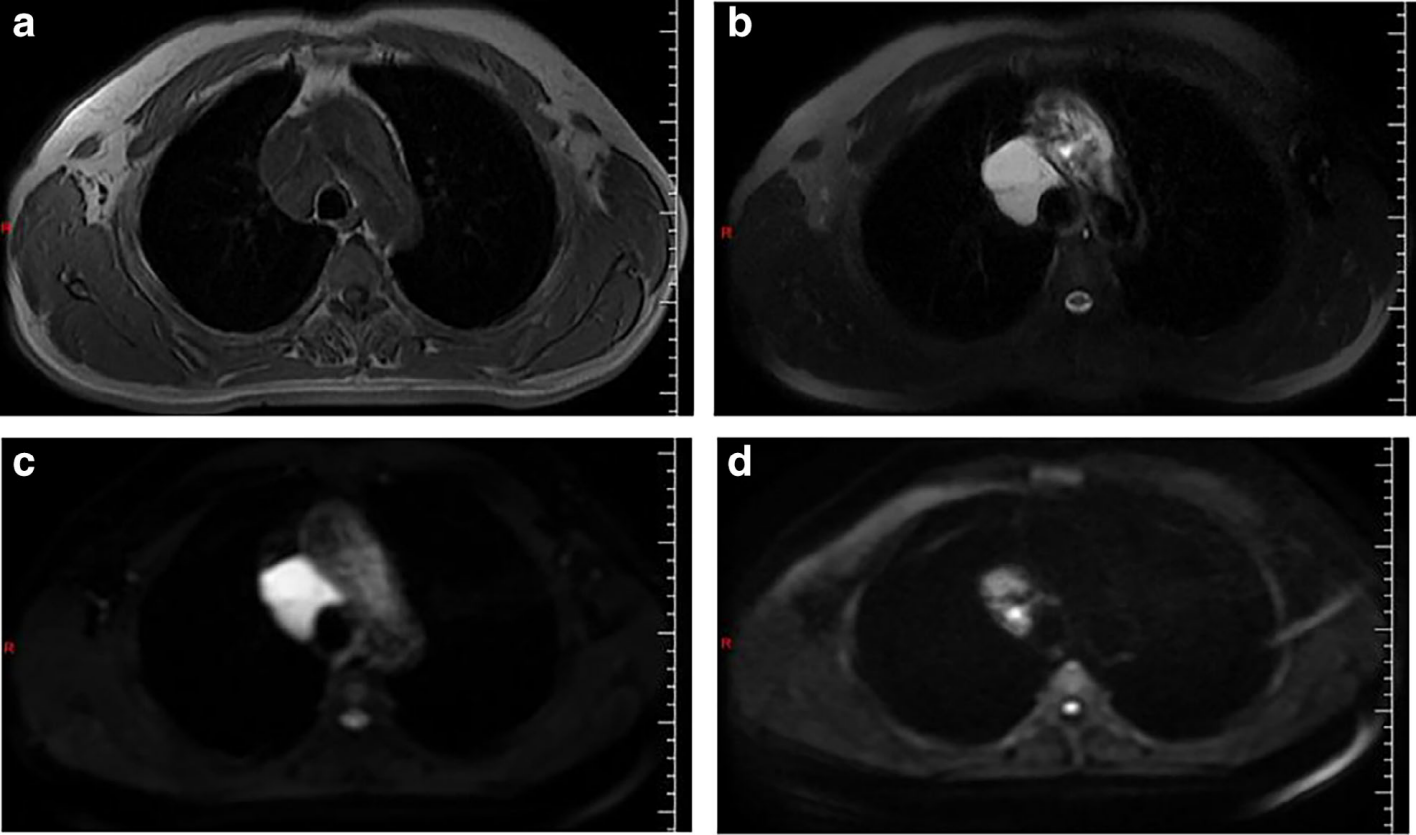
Irregular abnormal signals were seen in the vena cava‐anterior trachea space on chest MRI (a) with uniformly low signals on T1WI (b) and significantly high signals on T2WI. There was no obvious diffusion limitation of lesions on different b‐value DWI. (c,d) The lesion considers vascular lesion.
